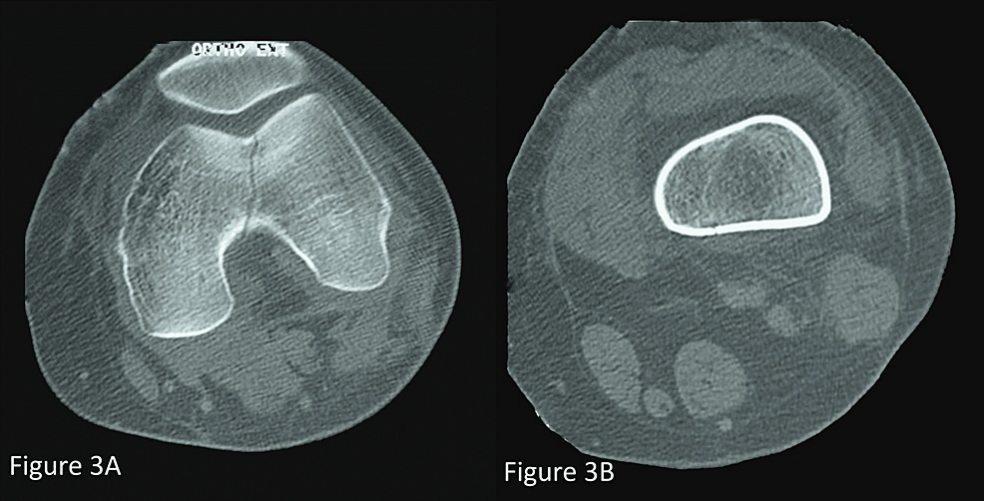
CT scan showing an intercondylar fracture without cortical damage or displacement
